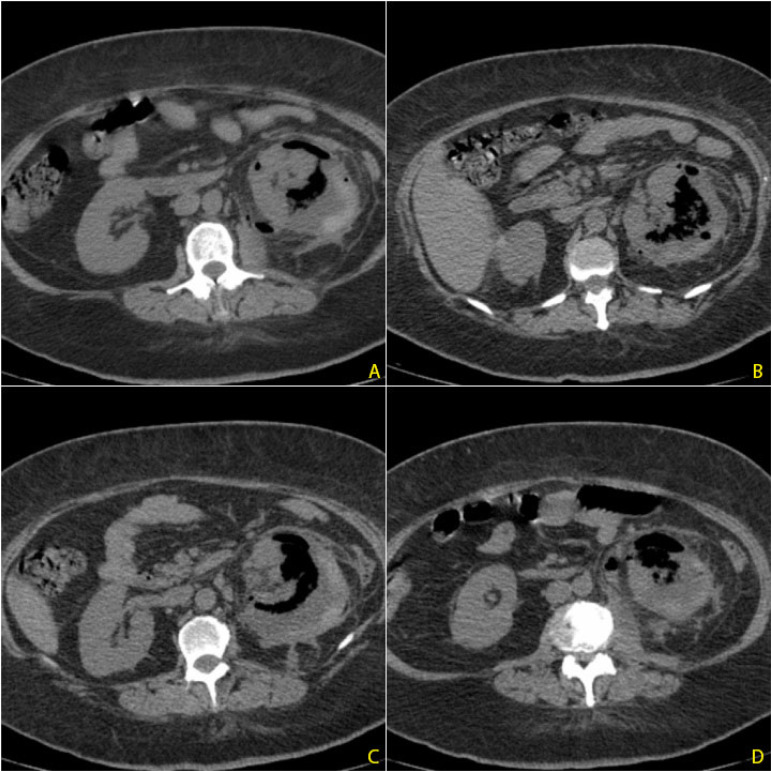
Computed tomography images of the abdomen without contrast. Panels A
